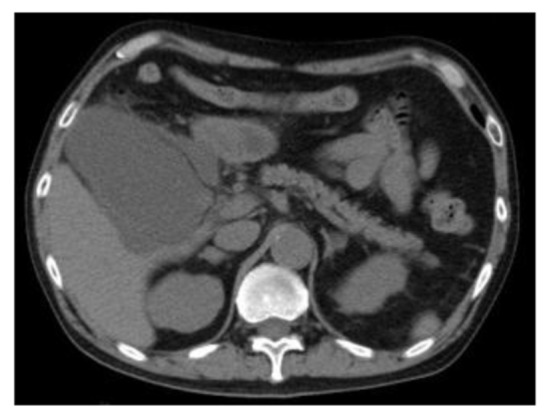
Abdominal computed tomography showed a tense and dilated gallbladder with surrounding fluid collection without gallbladder stones or emphysematous changes.
